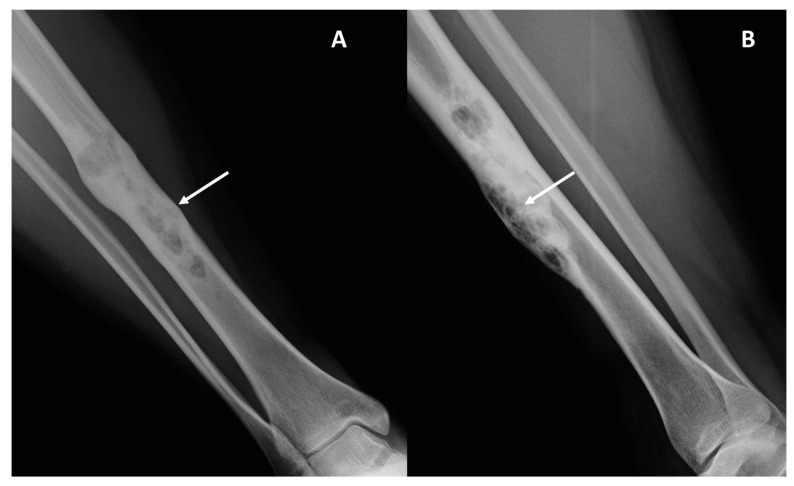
Conventional radiography (panel (A), antero-posterior view; panel (B), latero-lateral view) of the leg in a 38-year-old female with adamantinoma of the right tibial diaphysis. A mainly lytic geographic lesion with partial sclerotic borders and a “soap bubble” appearance (arrows) is displayed.
